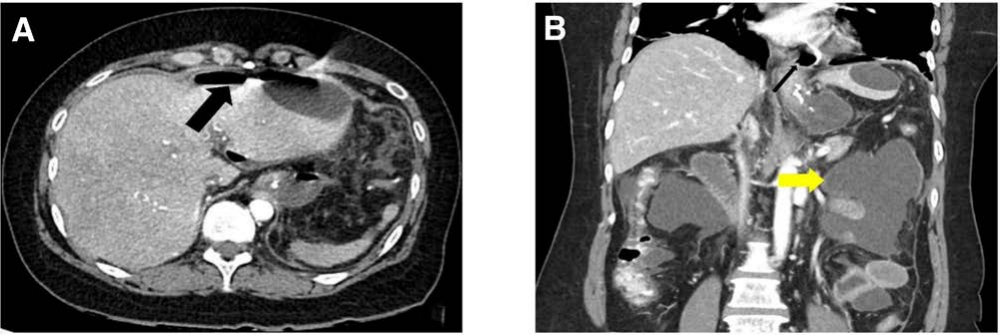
The second abdominal computed tomography scan. (A) The black arrow indicates the presence of free air. (B) The yellow arrow indicates an excessive intra-abdominal fluid collection, with associated wall enhancement, as observed in the frontal view.
